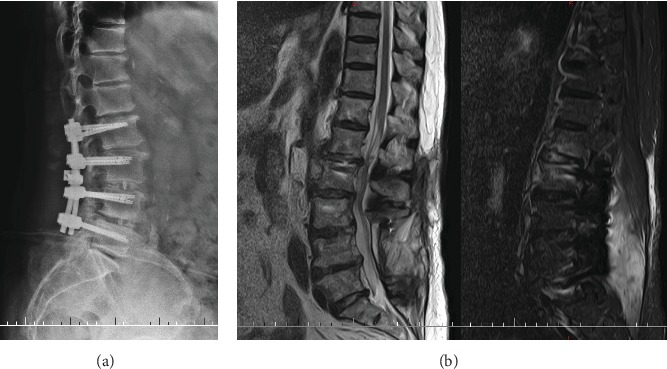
Lateral lumbar radiograph of second hospitalization showing no significant changes at the adjacent L1-2 segment comparing with one-month follow-up (a). Sagittal MRI T2-weighted and short-tau inversion recovery (STIR) images of second hospitalization showing inflammatory edema signal in intervertebral disc and bone marrow below the endplates of L1-2 (b).
